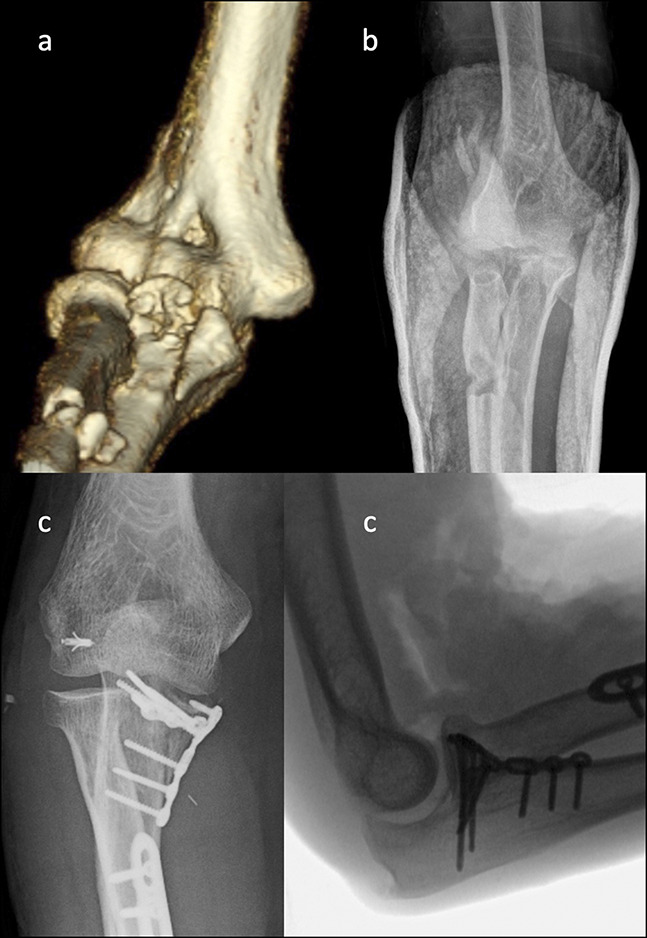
Three-dimensional CT scan (A) and radiology image (B) demonstrating anteromedial coronoid fracture. Radiographs demonstrating surgical fixation using a coronoid plate and repair of the lateral collateral ligament (C).
